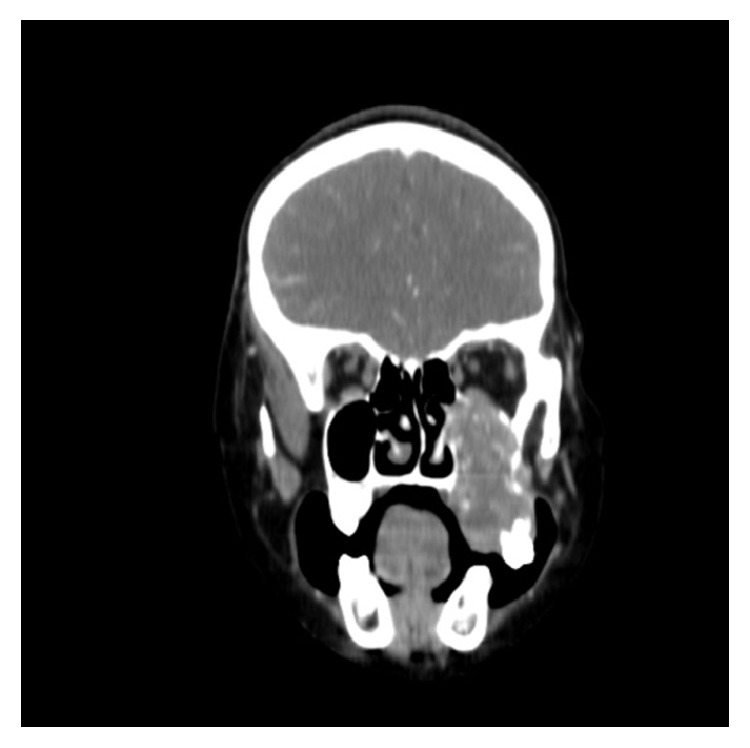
Coronal section of CT scan showing extent of tumor.
